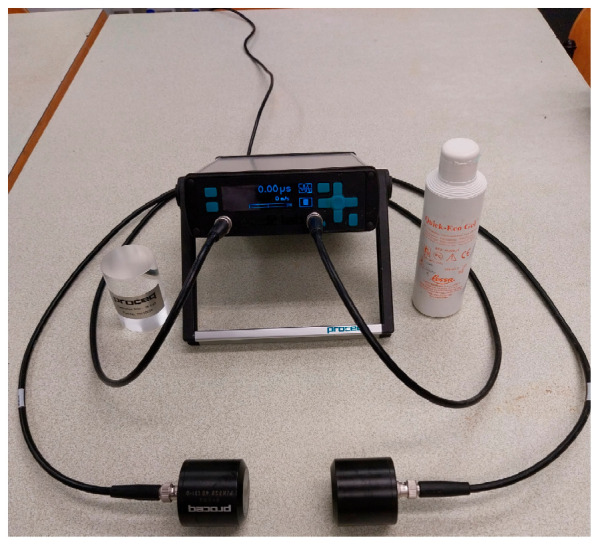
Equipment used for Ultrasonic Pulse Velocity by Wave Transmission (UPV) test.
